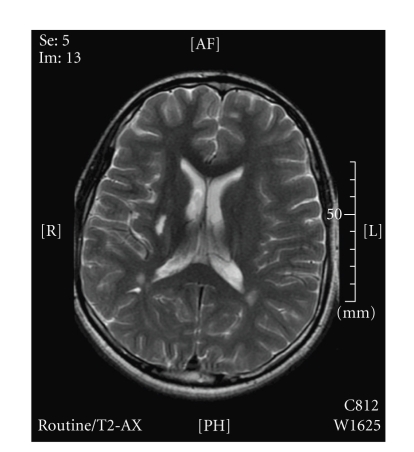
MRI 3 month after the initial insult showing a residual hyperdense signal abnormally in the periventricular region.
